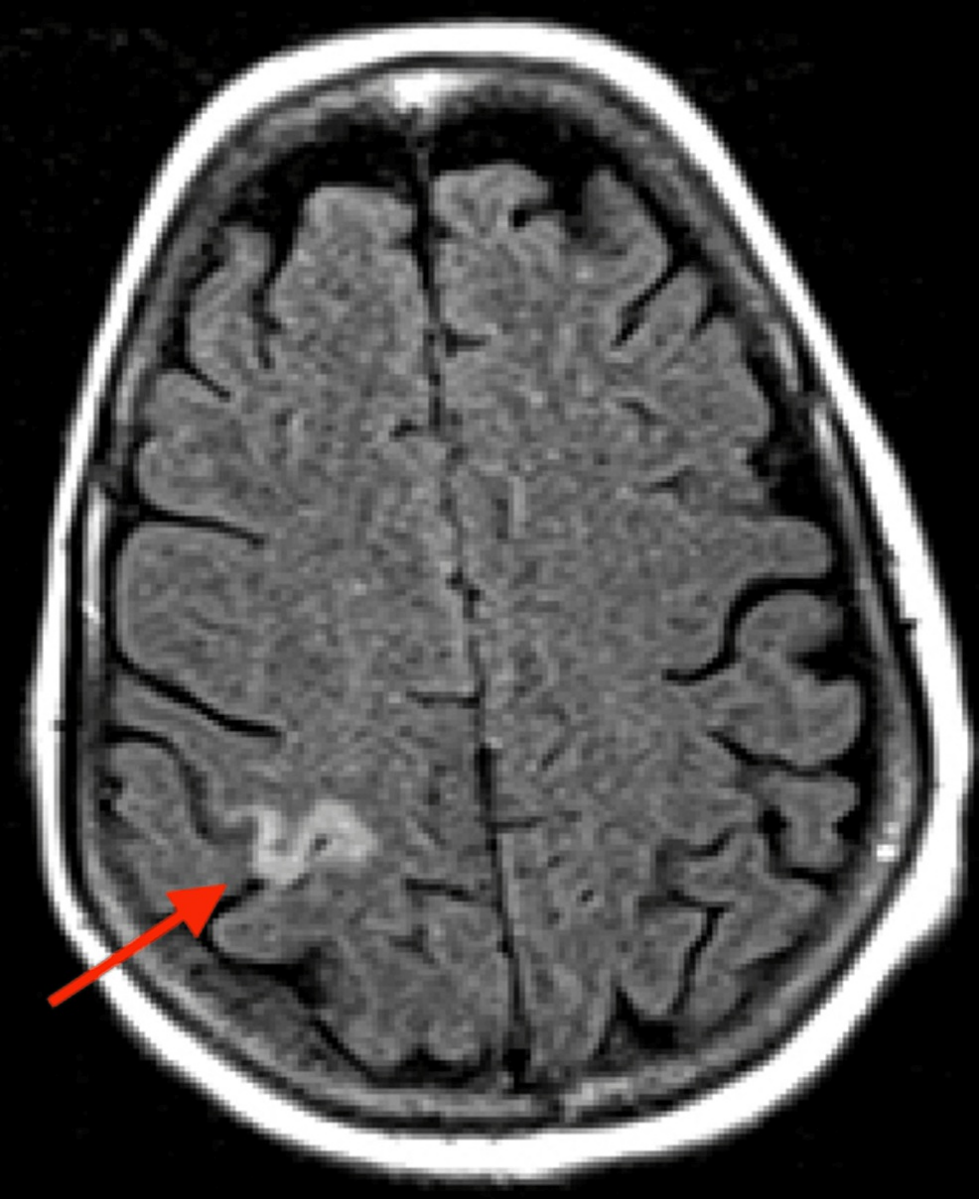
MRI axial flair, acute infarct involving high right parietal subcortical white matter.
